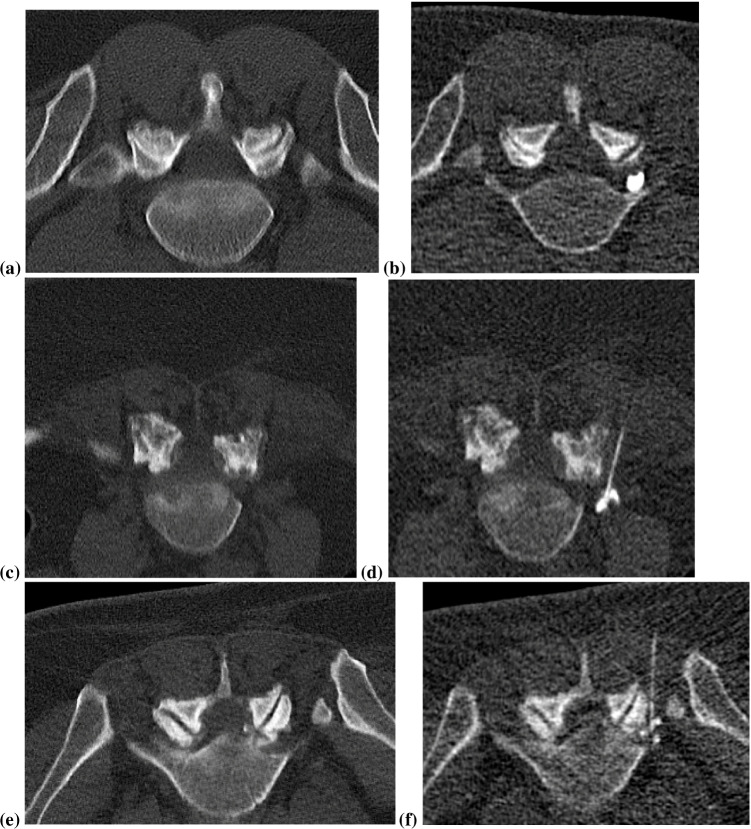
Examples of different contrast medium dispersal patterns in three different patients with right-sided periradicular L5 nerve root infiltration. On the left side of each row, the planning helical CT, on the right side the navigation CT-fluoroscopy image for needle placement, with more image noise due to the use of a low radiation dose CT protocol. a, b and c, d Focal non-linear contrast dispersal pattern. e, f Tram track contrast medium dispersal pattern
